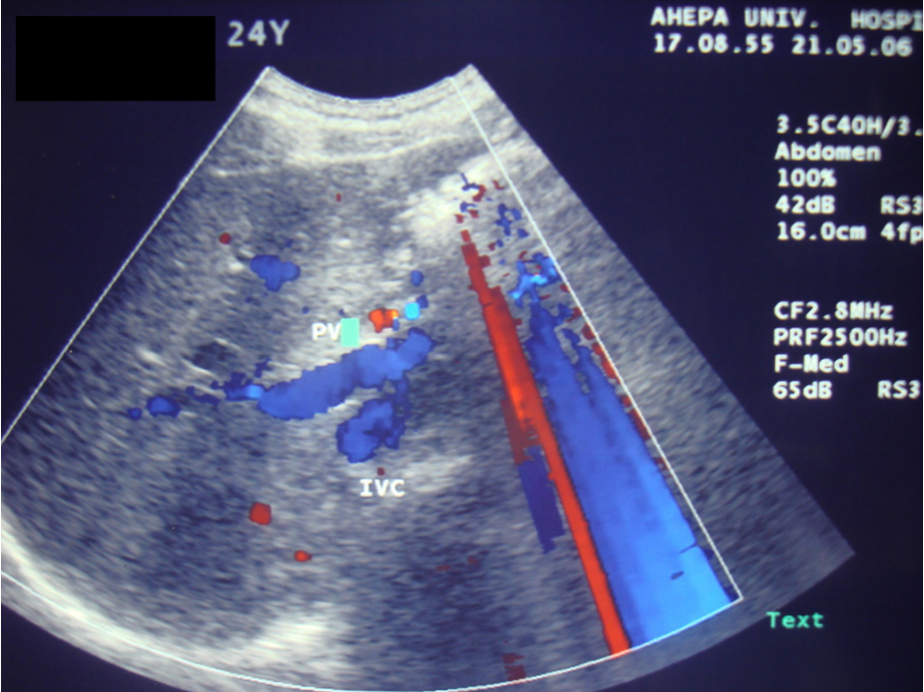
Ultrasonography showing dilated splenic vein of our patient.
